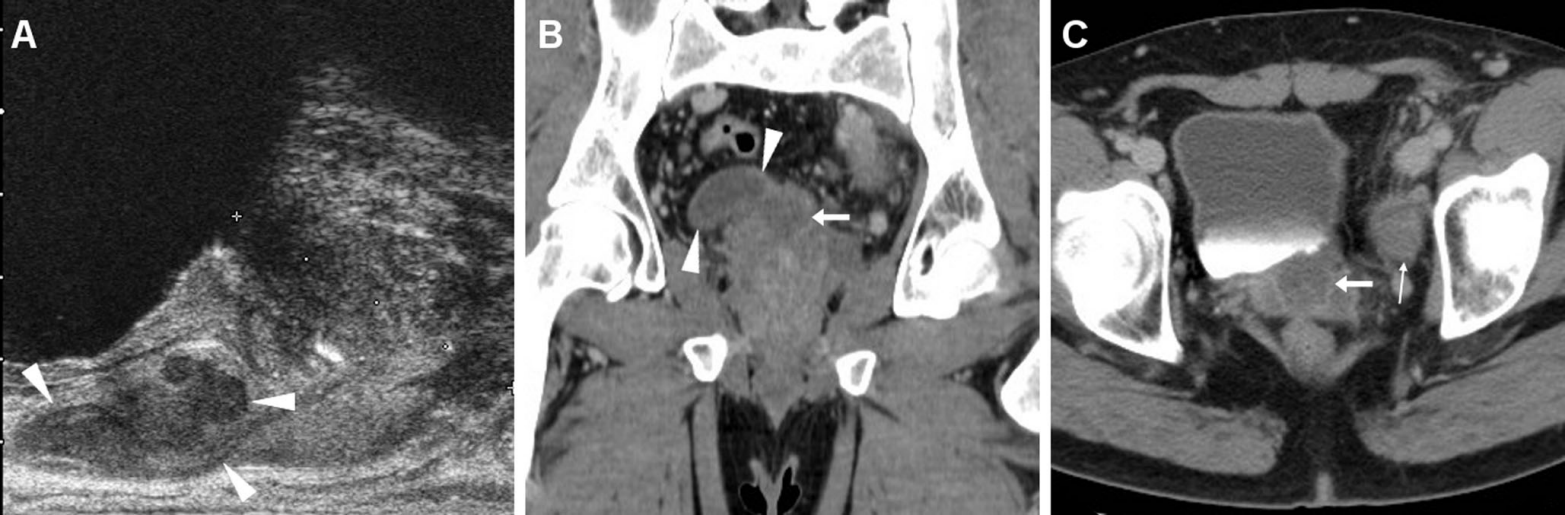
Chronic seminal vesicle infection in a 50-year-old HIV-positive man with haemospermia, appearing as hypoechoic enlargement (*arrowheads* in a) at transrectal ultrasound. CT urography (b, c) depicted a corresponding non-enhancing hypoattenuating “sac-like” structure with loss of normal septation (*arrowheads* in b), plus a focal hypoenhancing region at the prostatic base (*arrows*) and associated ipsilateral lymphadenopathy (*thin arrow*)
